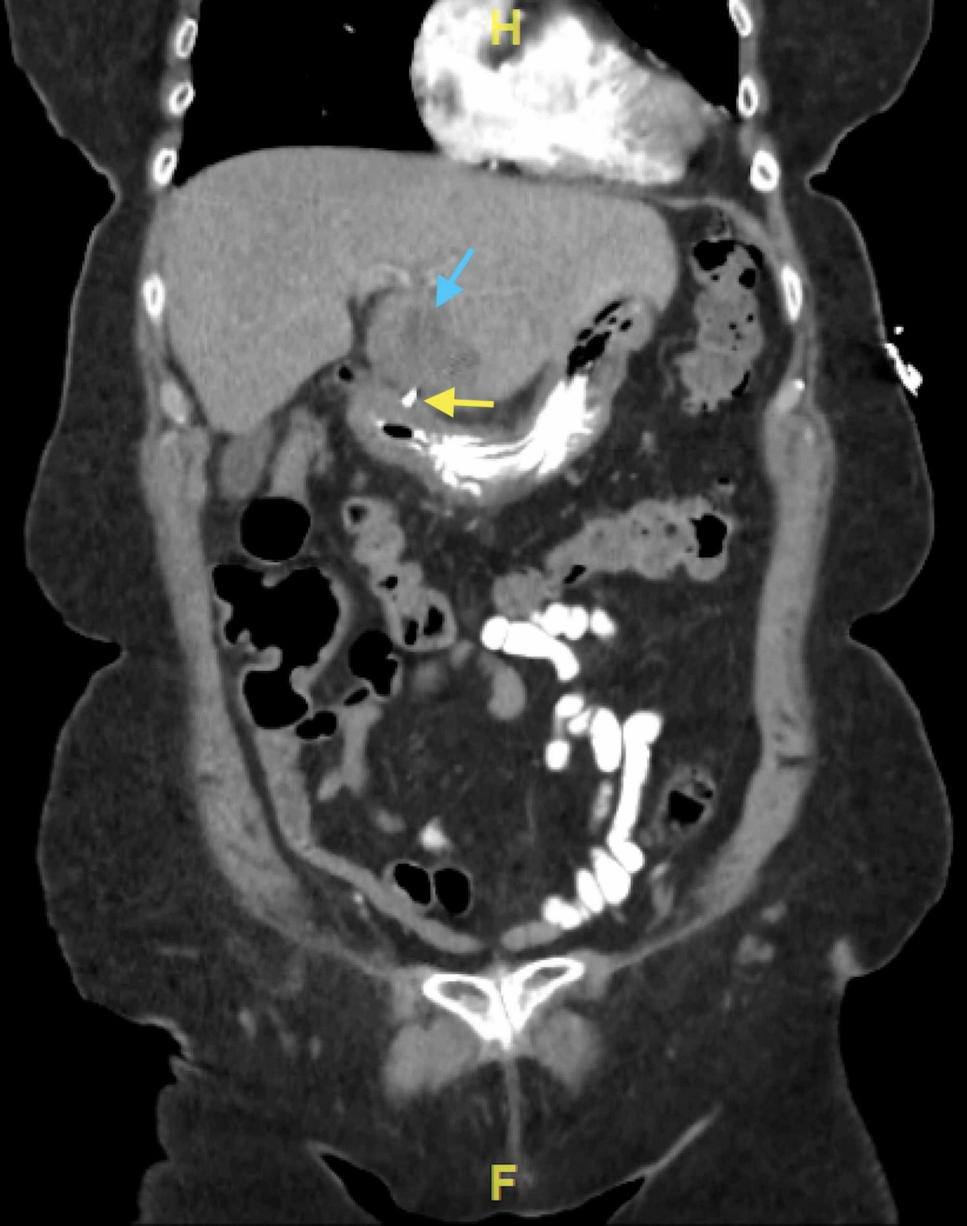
A coronal view of CT abdomen and pelvis showing a metallic foreign body (yellow arrow) penetrating the gastric antrum into a hypodense structure in the liver (blue arrow)
